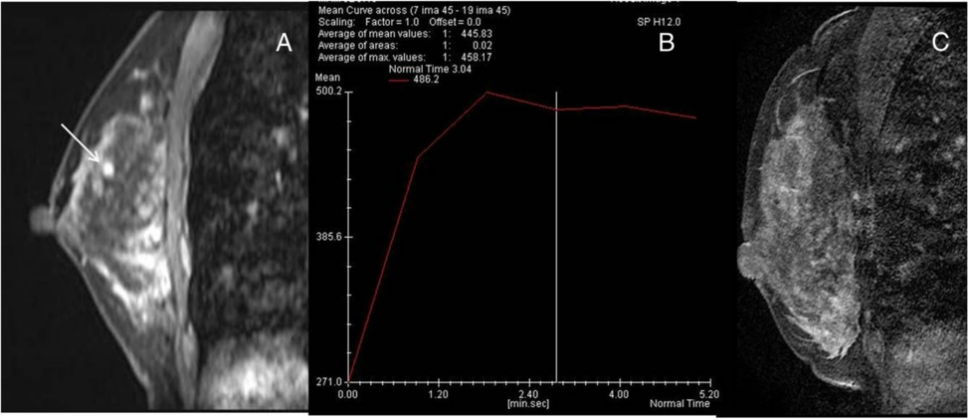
A 38-year-old woman with breast cancer on preoperative magnetic resonance imaging for staging. (A) Sagittal, contrast enhanced T1-weighted image showing oval, smooth, heterogeneous enhancing mass in the right breast, measuring 6 mm, which was categorized into American College of Radiology Breast Imaging Reporting and Data System (BI-RADS) category 4a. (B) Dynamic time-intensity curves of the lesion showing an initial fast uptake followed by a fast washout in the delayed phase (type III), which was categorized into BI-RADS category 4a. (C) Sagittal, contrast enhanced T1-weighted image on the day of biopsy showing no suspicious lesions, and hence magnetic resonance imaging-guided breast biopsy was cancelled.
